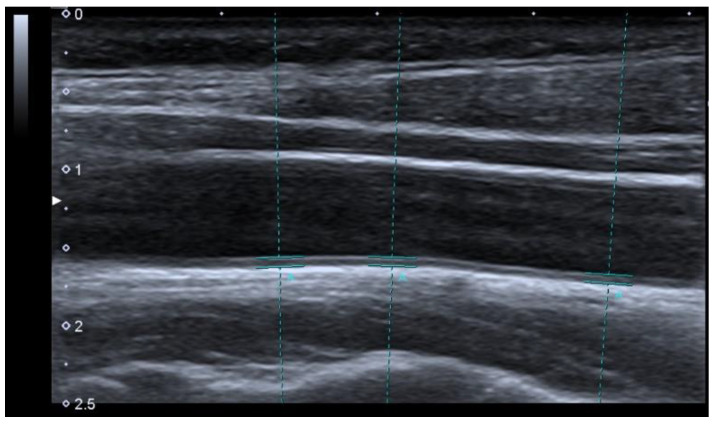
Intima-media thickness *ultrasound measurement* (A).
